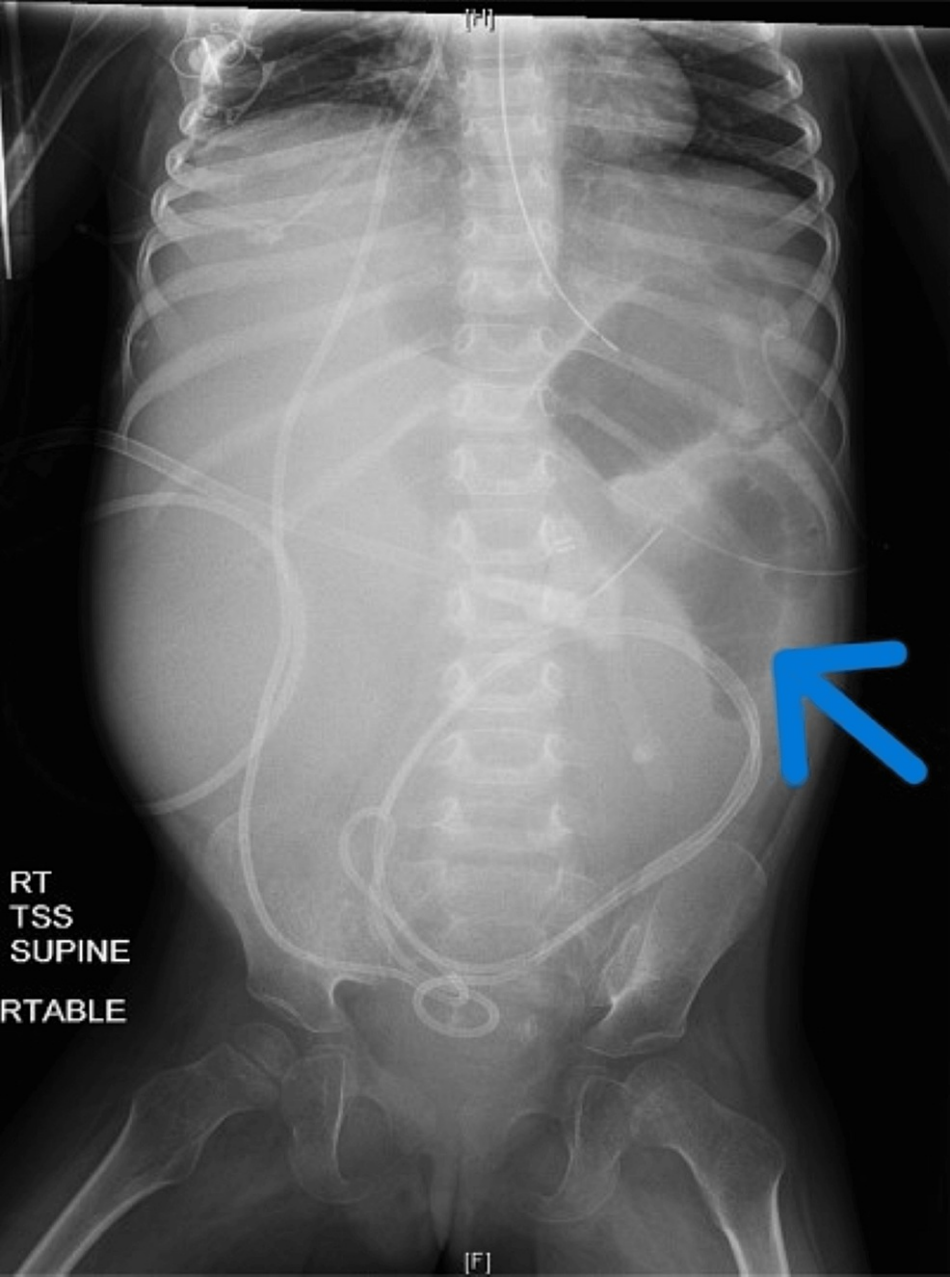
Repeat abdominal X-ray revealing persistent evidence of bowel gas paucity and bowel loop distention as identified by blue arrow
